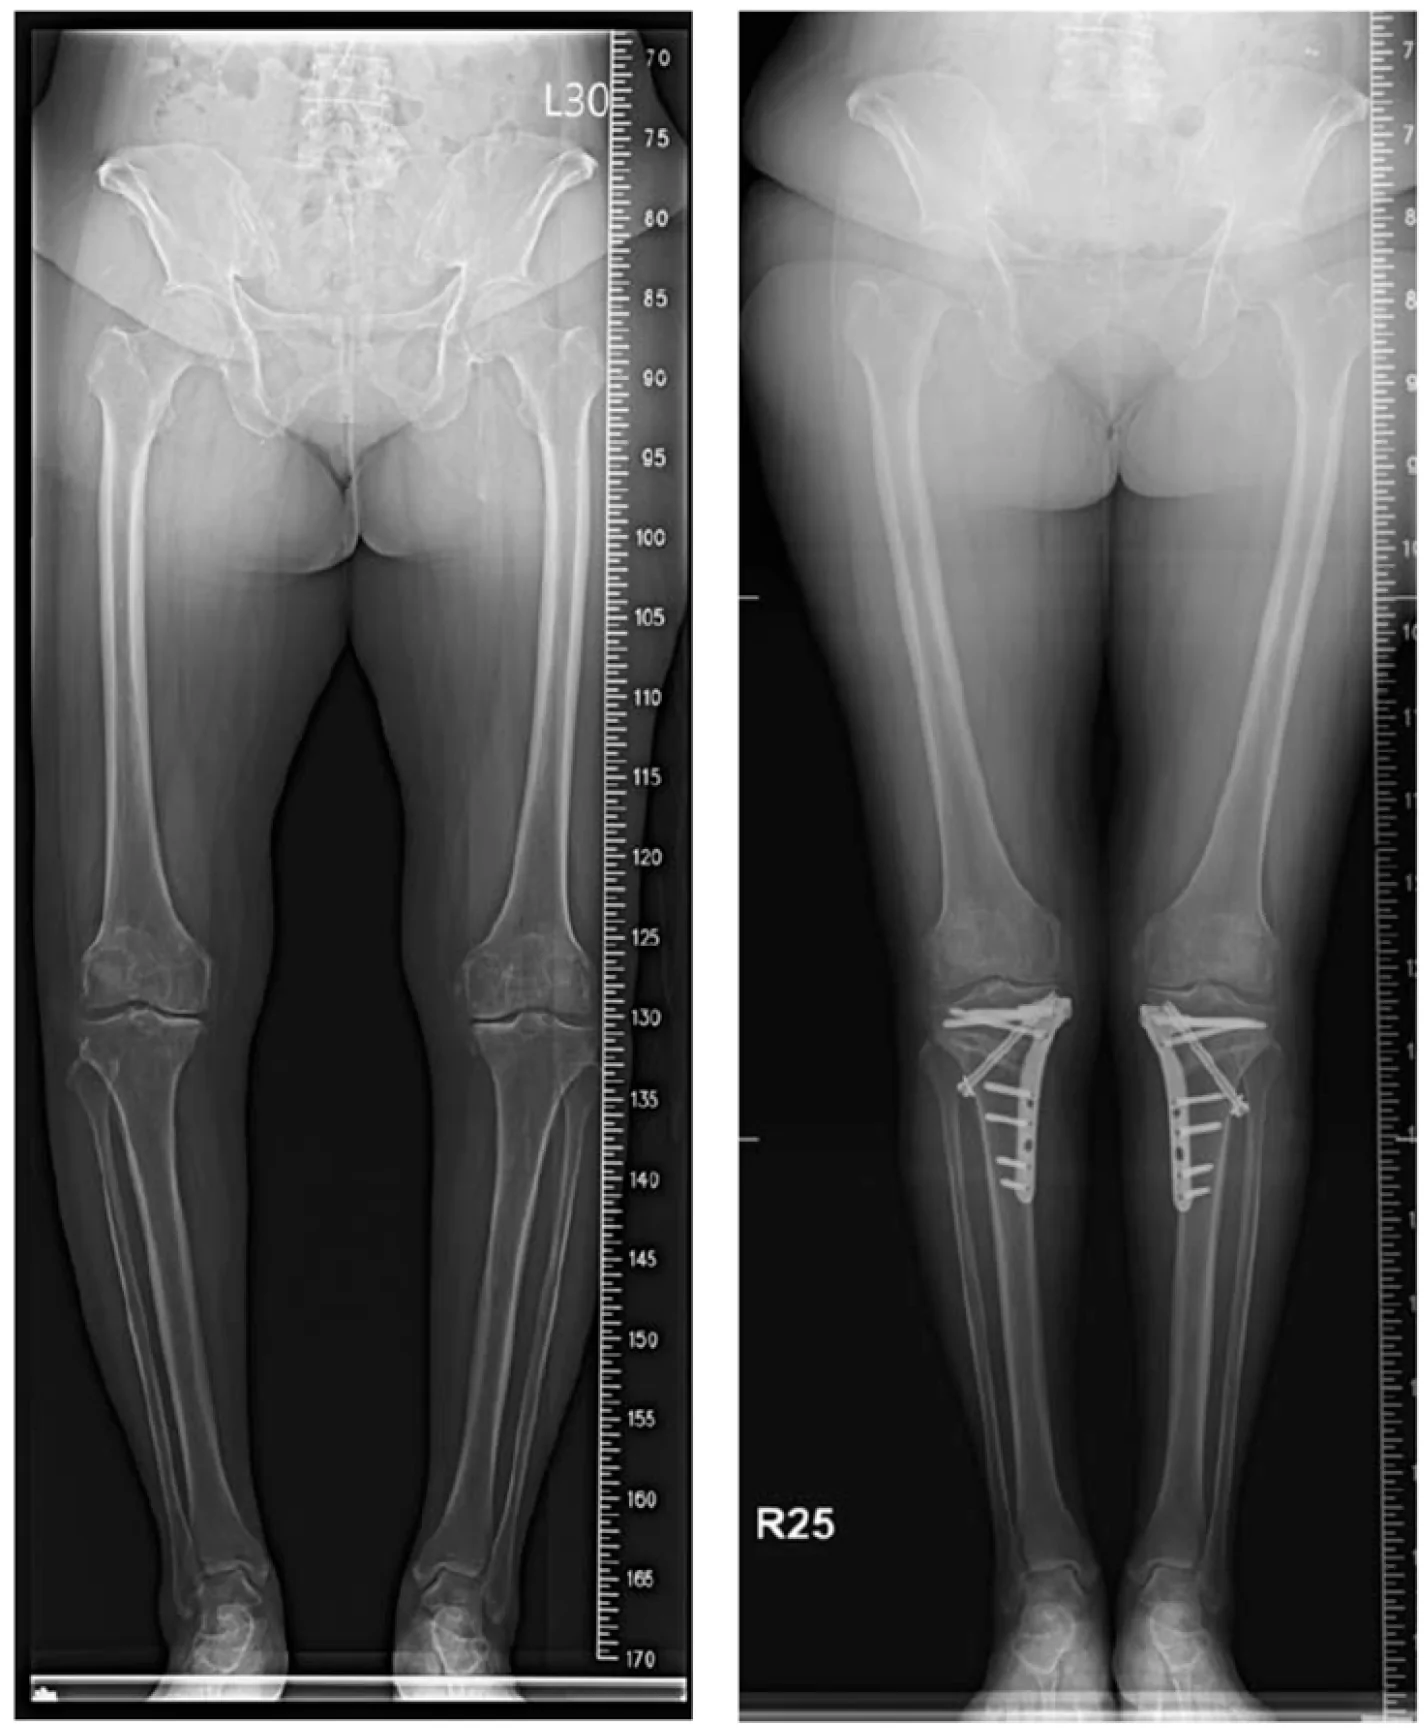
X-ray of opening-wedge valgus HTO. The left image is an X-ray taken before the surgery, and the right image is an X-ray taken after the surgery.
